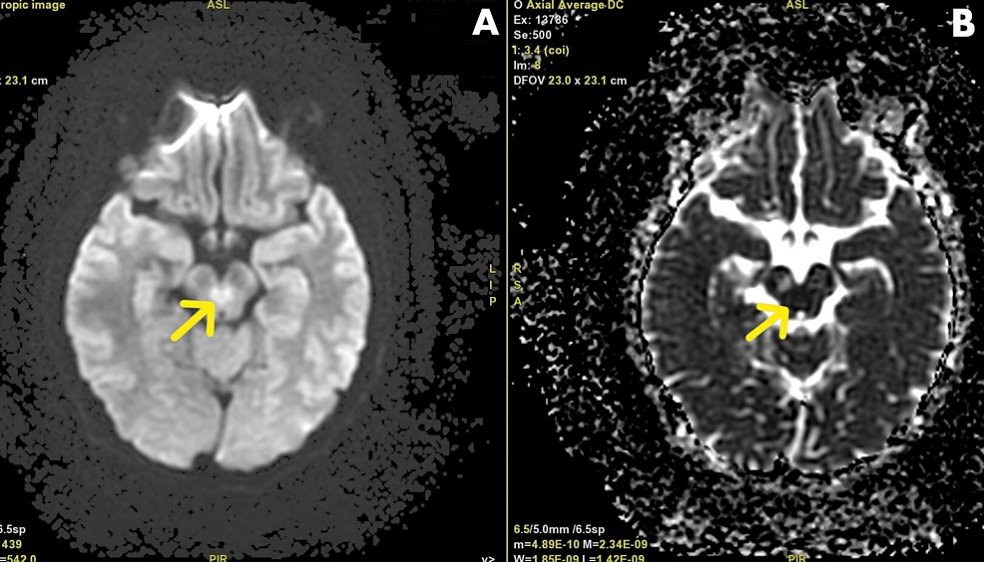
Brain MRI of the patient showing (A) an area of hyperintensity seen in bilateral tegmentum in DWI sequence and (B) appearing hypointense in ADC mapping, suggestive of Wernekink commissure syndrome. The arrows in both panes A and B point to the areas showing the features mentioned in the corresponding panes. MRI, magnetic resonance imaging; DWI, diffusion-weighted imaging; ADC, apparent diffusion coefficient
